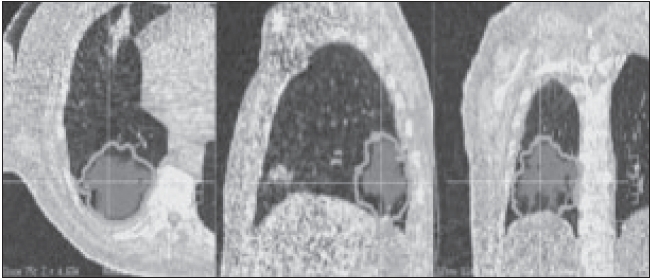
Motion envelope derived by deformable image registration. The area in color wash is the PTVex (original PTV excluding breathing motion). Contours show the envelope on the treatment planning CT images (at full exhalation phase)
